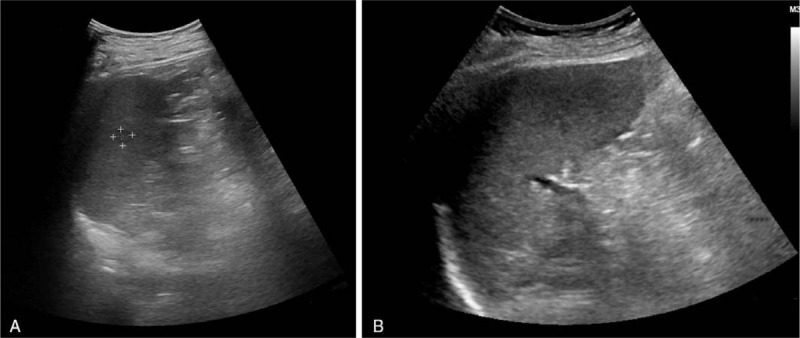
Contrast of spleen ultrasound images. (A) Ultrasound of intrasplenic nodule. (B) Splenomegaly ultrasonography.
